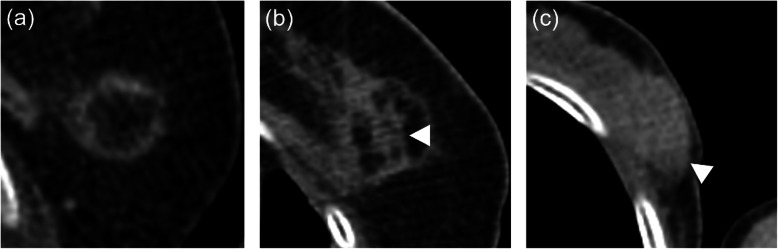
Classification of CT findings on ^18^F-FDG PET/CT after breast-cryoablation. CT findings were classified as fatty mass type (a, b) or non-fatty mass type (c). Fatty mass type was defined as the treated area including fat density, including a fatty mass with a soft tissue density septum (b, arrowhead). Non-fatty mass type was defined as the treated area consisting only of soft tissue density (c, arrowhead)
